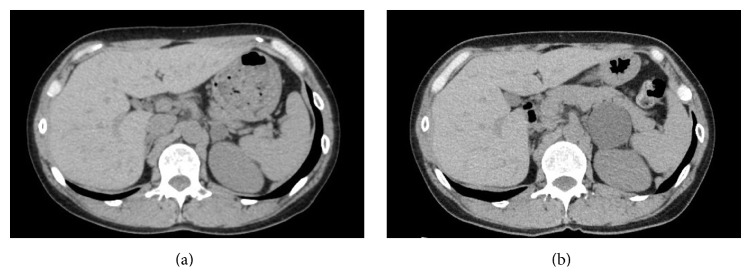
Unenhanced computed tomography of the abdomen showing a unilocular, noncalcified, hypoattenuating left adrenal lesion. The lesion increased in size from (a) 10 mm to (b) 50 mm at two-year follow-up.
